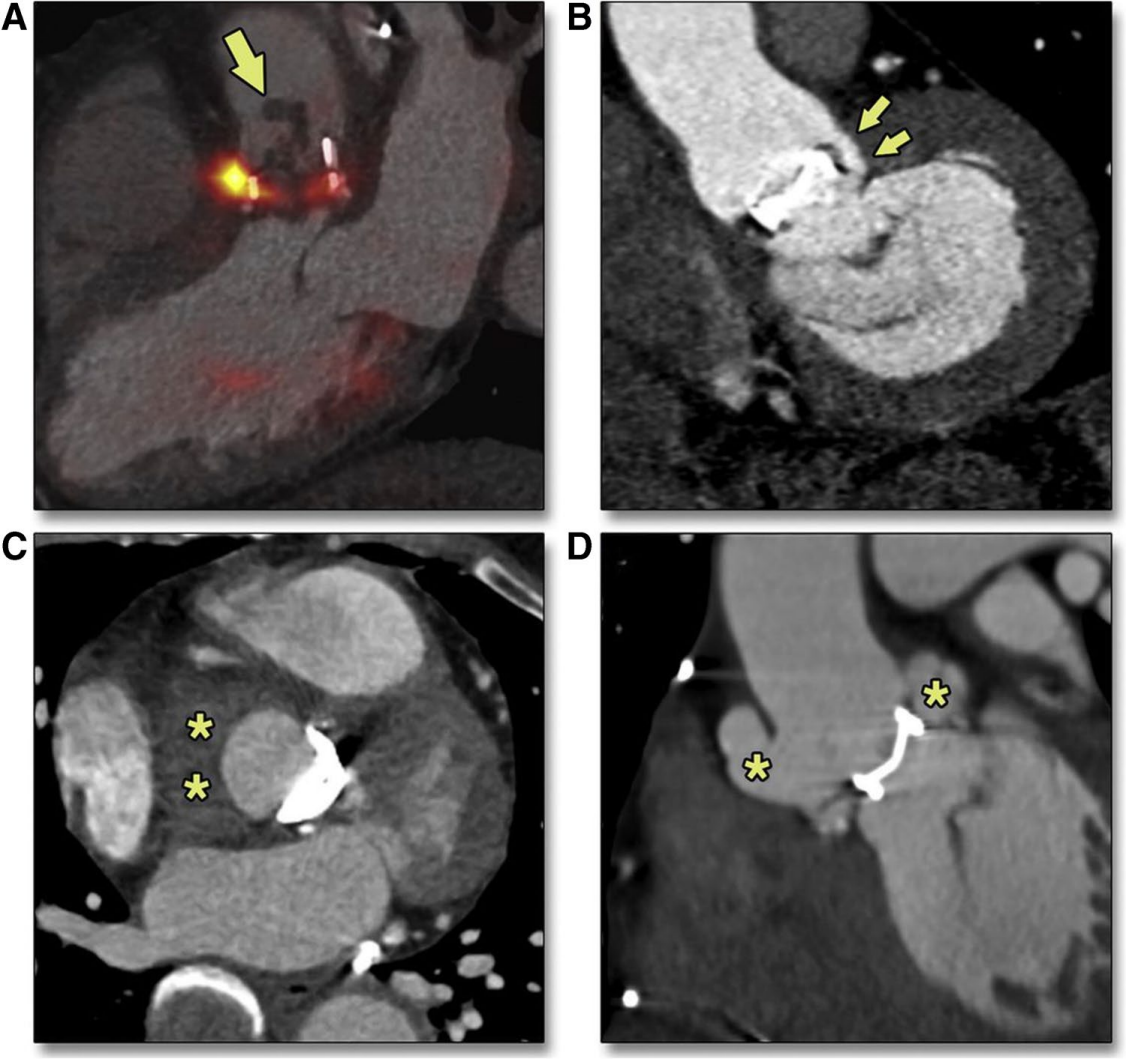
A Large aortic vegetation (arrow) in late (3 years post-implantation) prosthetic aortic valve endocarditis, blood culture–positive for Staphylococcus epidermidis. (B) Paravalvular fistula (arrows) in late (15 years post-implantation) prosthetic aortic valve endocarditis, culture-positive for Streptococcus bovis. (C) Poorly delimited perivalvular soft tissue mass (asterisks) corresponding to a periprosthetic abscess in a patient with infective endocarditis (9 years post-implantation), culture-positive for Enterococcus faecalis. (D) Multilobulated periprosthetic pseudoaneurysm (asterisks) in a patient with relapsing endocarditis (11 months post-implantation), culture-positive for Mycobacterium avium intracellulare. CTA = computed tomography angiography. Reprinted with permission of Elsevier from [79]
